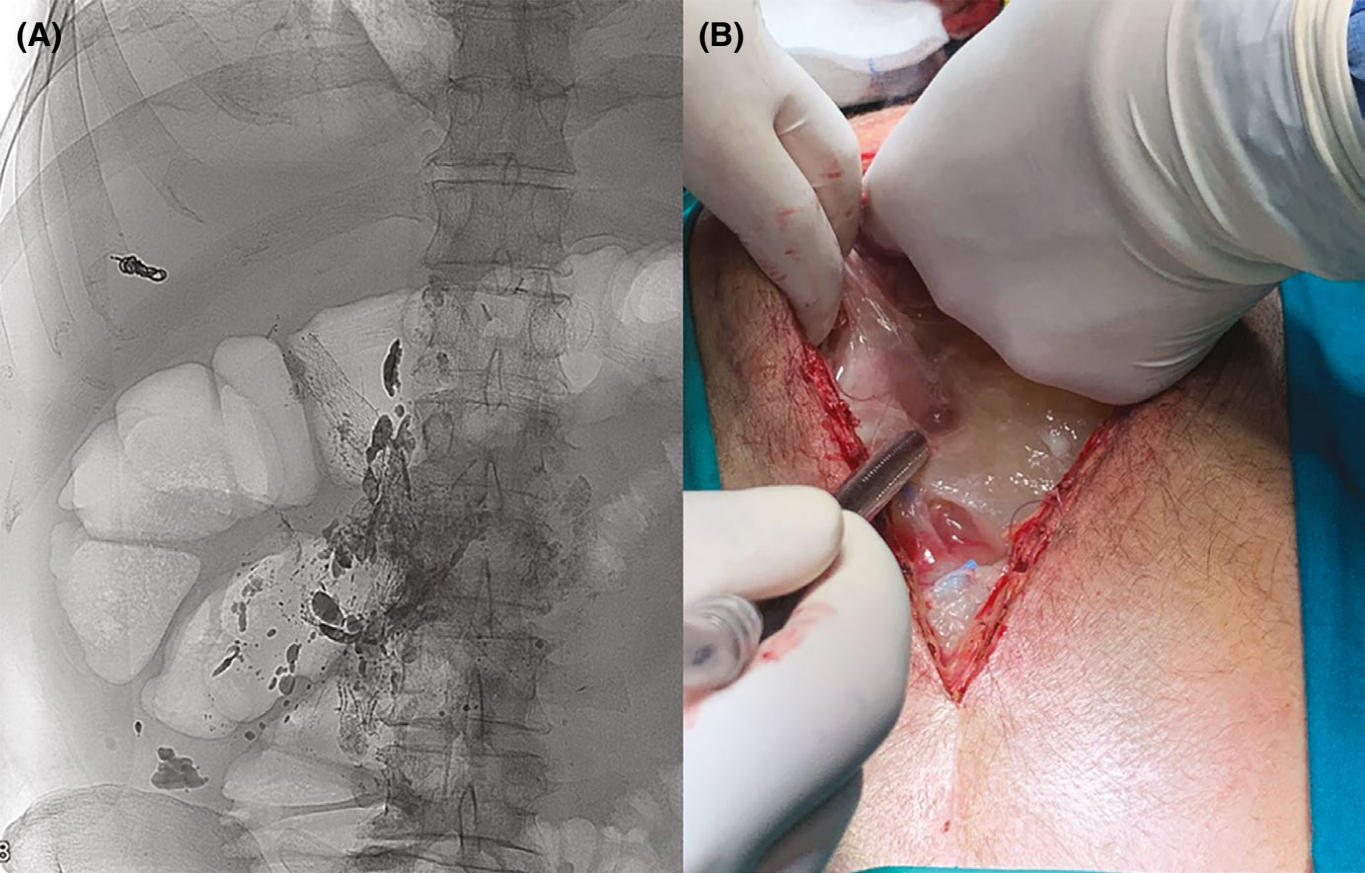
A, Lymphoscintigraphy and lymphangiogram showing a chyle leak adjacent to cisterna chyli. B, Intraoperative finding of chylous ascites with dense adhesions
